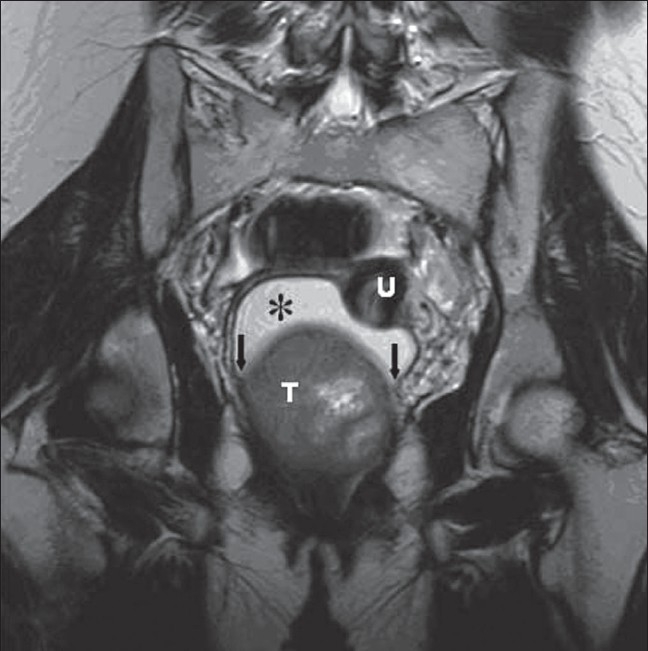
Coronal T2W (TR / TE = 5000 / 100) MRI shows the mass (T), displacing the uterus (U) cranially, and causing distension of the fornices and enlargement of the vaginal lumen* with high signal intensity. Note the acute angles (arrows) between the tumor and vaginal wall, suggesting its vaginal origin
